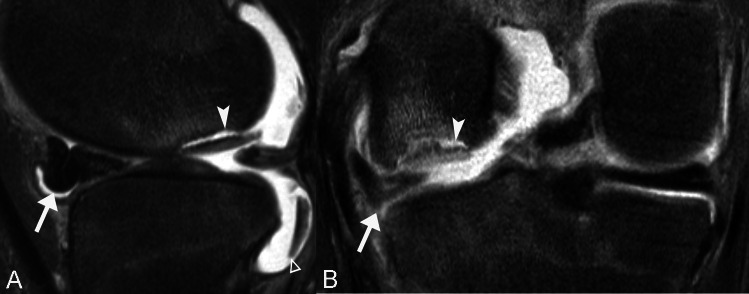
Unstable LFC OCD lesion in a 17-year-old skeletally mature female. Sagittal T2-weighted fat-suppressed (A) and coronal intermediate-weighted fat-suppressed (B) MR images show a partially detached progeny with a fluid-signal intensity progeny-parent bone interface (arrowheads). Note the intra-articular body posteriorly (triangle) and a torn, anteriorly shifted and laterally extruded discoid lateral meniscus (arrows). These findings were confirmed during arthroscopy.
